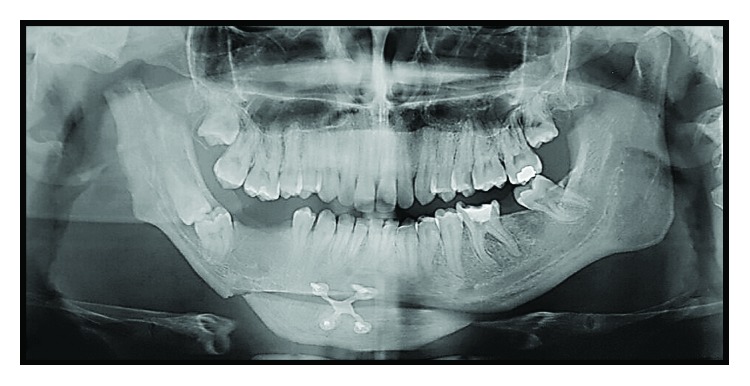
Panoramic radiography after seven days emphasizing the stable internal fixation of the chin and bone gap on the right side of the TMJ region.
